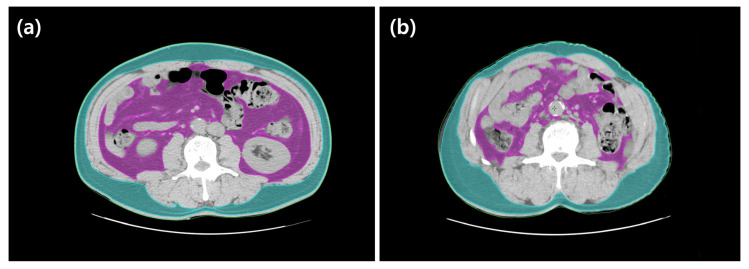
Representative computed tomography scans at the L3 level with identical body mass index (BMI) but varying visceral adipose tissue (VAT) area. (a) A patient with a BMI of 25.4 kg/m^2^ and a VAT area of 72.38 cm^2^, demonstrating a complete response. (b) A patient with an identical BMI of 25.4 kg/m^2^ and a VAT area of 16.82 cm^2^, demonstrating a partial response.
